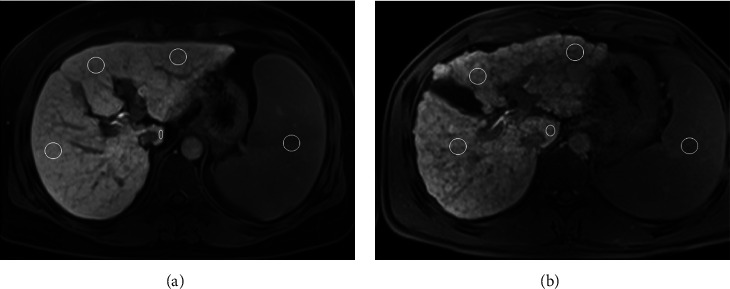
Two patients with hepatitis B-related cirrhosis in compensated (a) and decompensated (b) statuses have undergone gadolinium ethoxybenzyl diethylenetriamine pentaacetic acid-enhanced axial T_1_-weighted magnetic resonance imaging. Circles with areas of 1-2 cm^2^ on the 20 minutes hepatobiliary-phase magnetic resonance images are regions of interest used for the measurements of signal intensity of the right liver lobe, left medial liver lobe, left lateral liver lobe, caudate lobe, and spleen.
